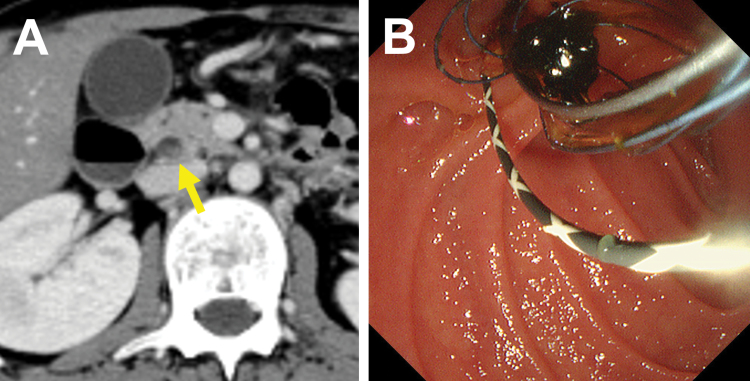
Development of choledocholithiasis during the clinical course. (A) CT image revealing a newly formed CBD stone. (B) Endoscopic image showing extraction of the CBD stone after endoscopic sphincterotomy. CBD, common bile duct; CT, computed tomography.
